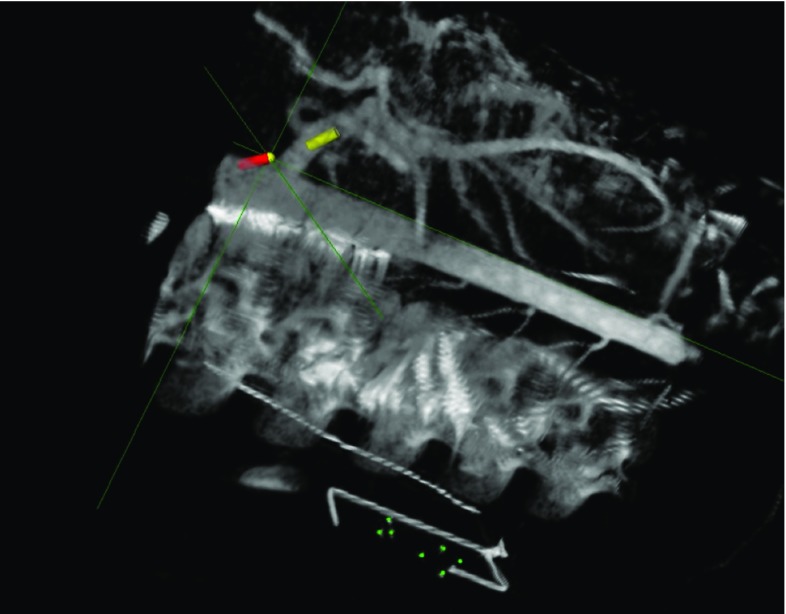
CustusX 3D view during endovascular navigation showing cone beam CT image volume and real-time position tracking of catheter (*red cylinder mark*) and guidewire (*yellow cylinder mark*)
